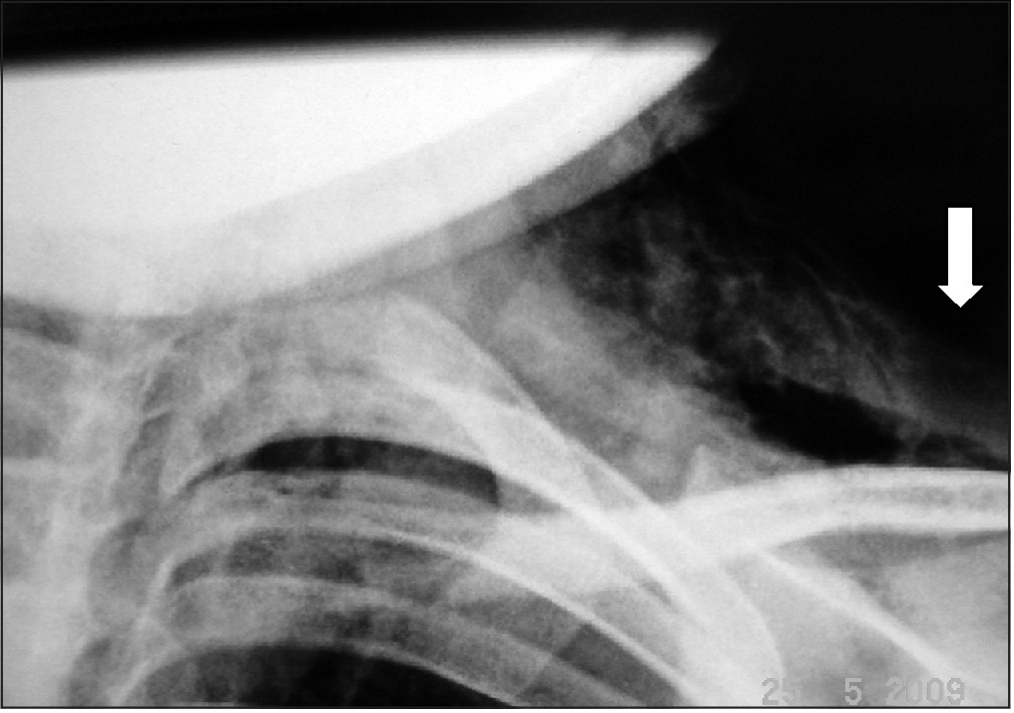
A closer view of the X-ray picture showing subcutaneous emphysema (white arrow)
